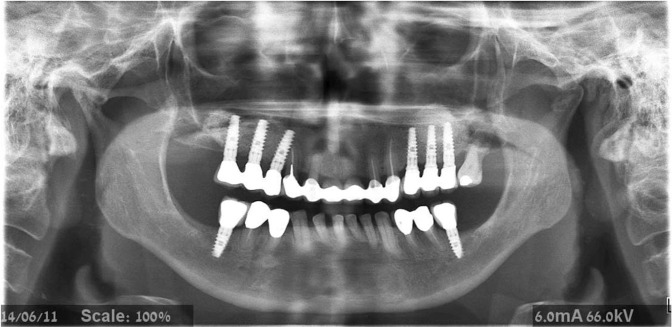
Panoramic radiograph demonstrating block-graft on the right side and particulate graft on the left side of the maxilla.
